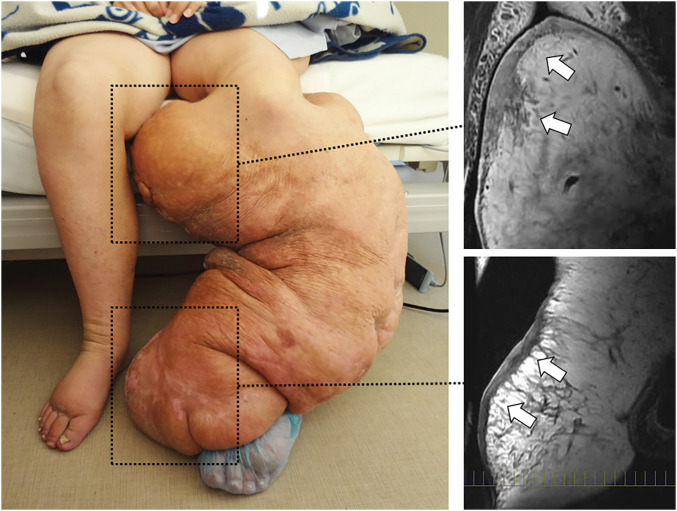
The left leg following aggressive decongestion. In the upper and lower medial calf, where hard and round protrusions were present (dotted rectangles), shrinkage could not be achieved with compression therapy. MRI revealed severe skin thickening (white arrows) in these areas.
